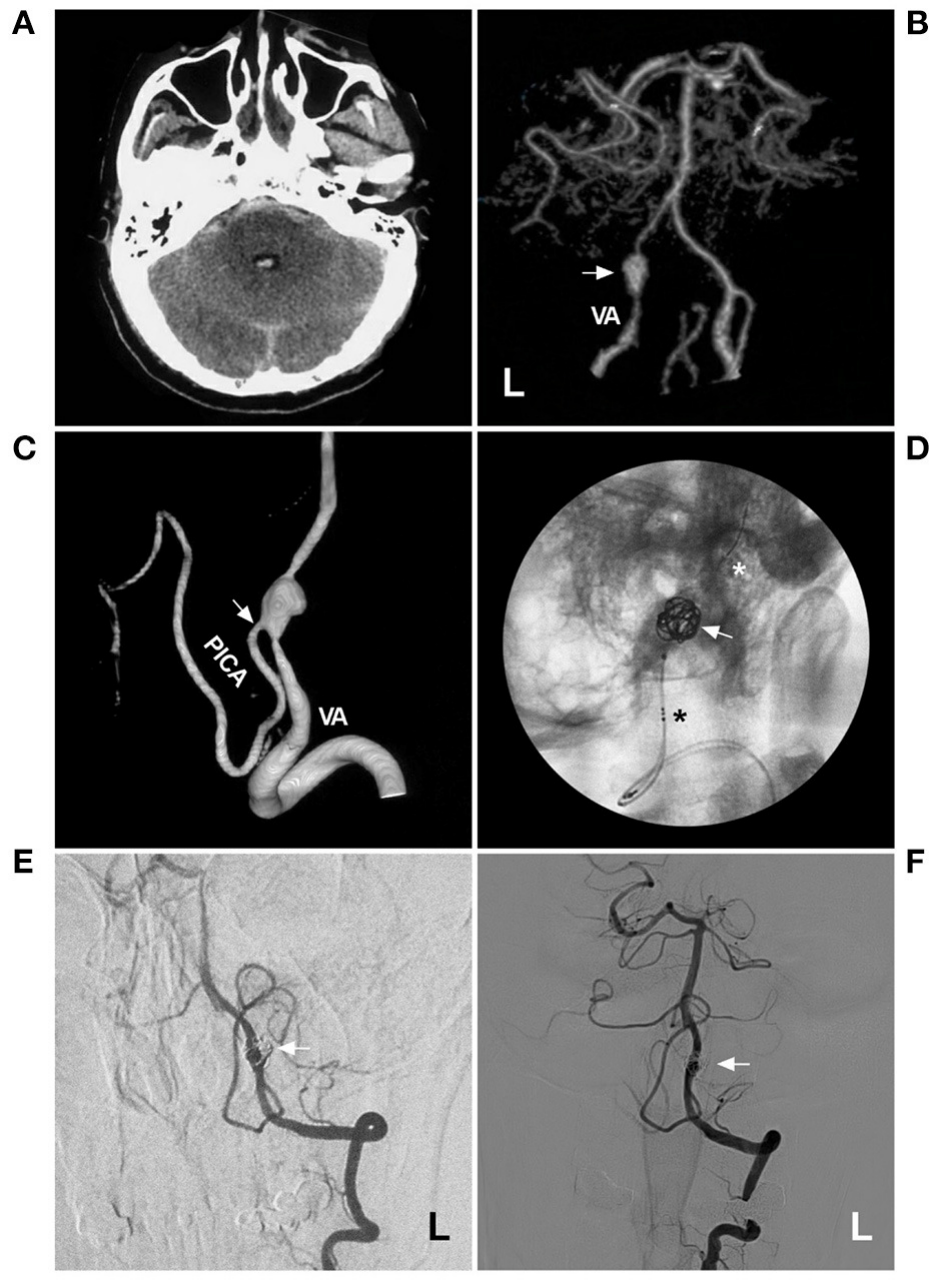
Coiling a VA acute fusiform aneurysm with stent assistance. (A) CT showed subarachnoid hemorrhage and fourth intraventricular hemorrhage. (B) CTA showed a fusiform aneurysm (arrow) on the left VA. (C) Three-dimensional DSA showed the PICA (arrow) from the aneurysm. (D) X-ray film showed the LVIS (asterisks indicate the proximal and distal markers) to assist in coiling the aneurysm. (E,F) 1-year (E) and 2-year (F) follow-up DSA of the left VA showed no recurrence of the aneurysm. CT, computed tomography; CTA, computed tomography angiography; DSA, digital subtraction angiography; L, left; PICA, posterior inferior cerebellar artery; VA, vertebral artery.
